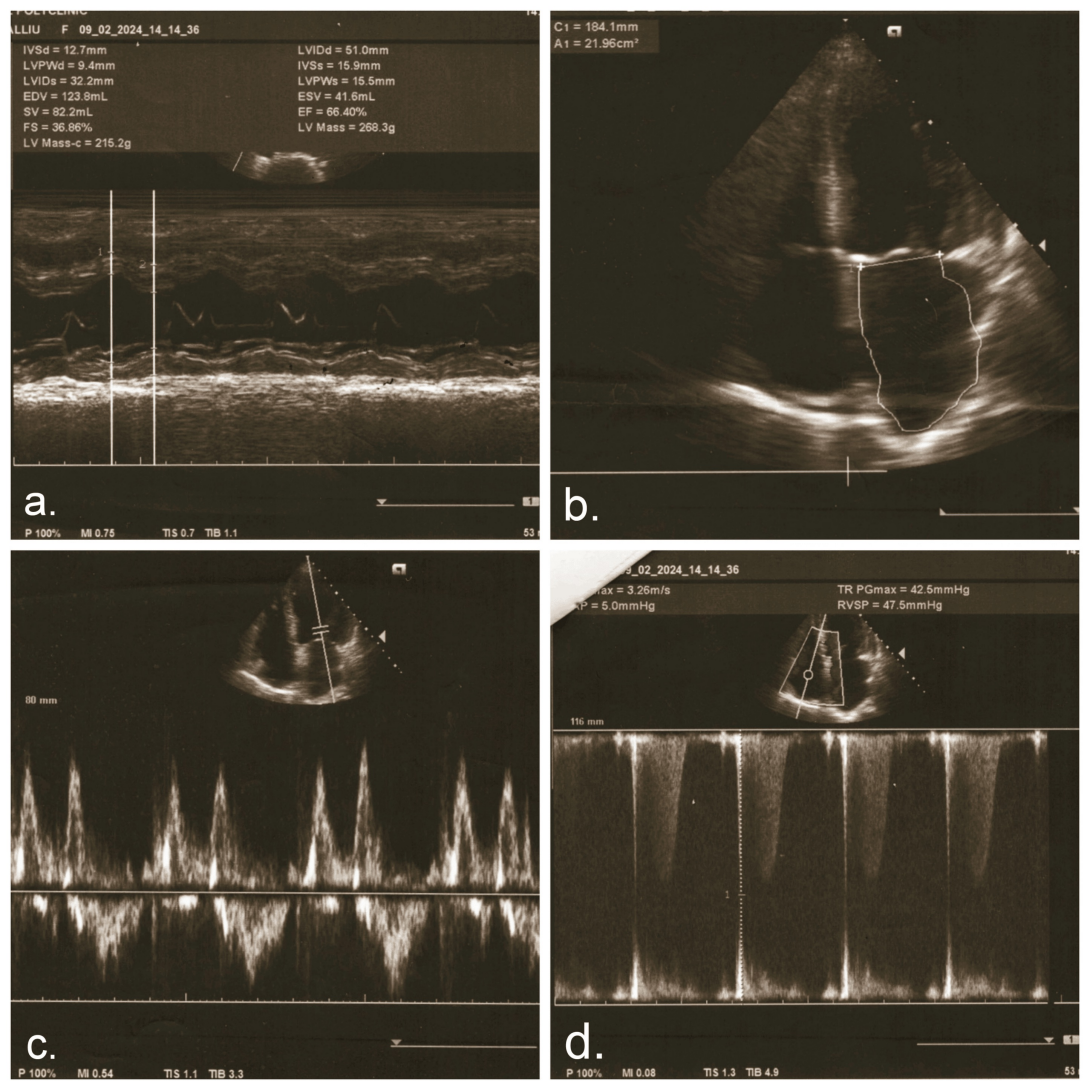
Cardiac ultrasound findings a: Normal ejection fraction. b: Left ventricular hypertrophy. c: Mitral valve regurgitation. d. Continuous wave Doppler assessment of tricuspid regurgitation
